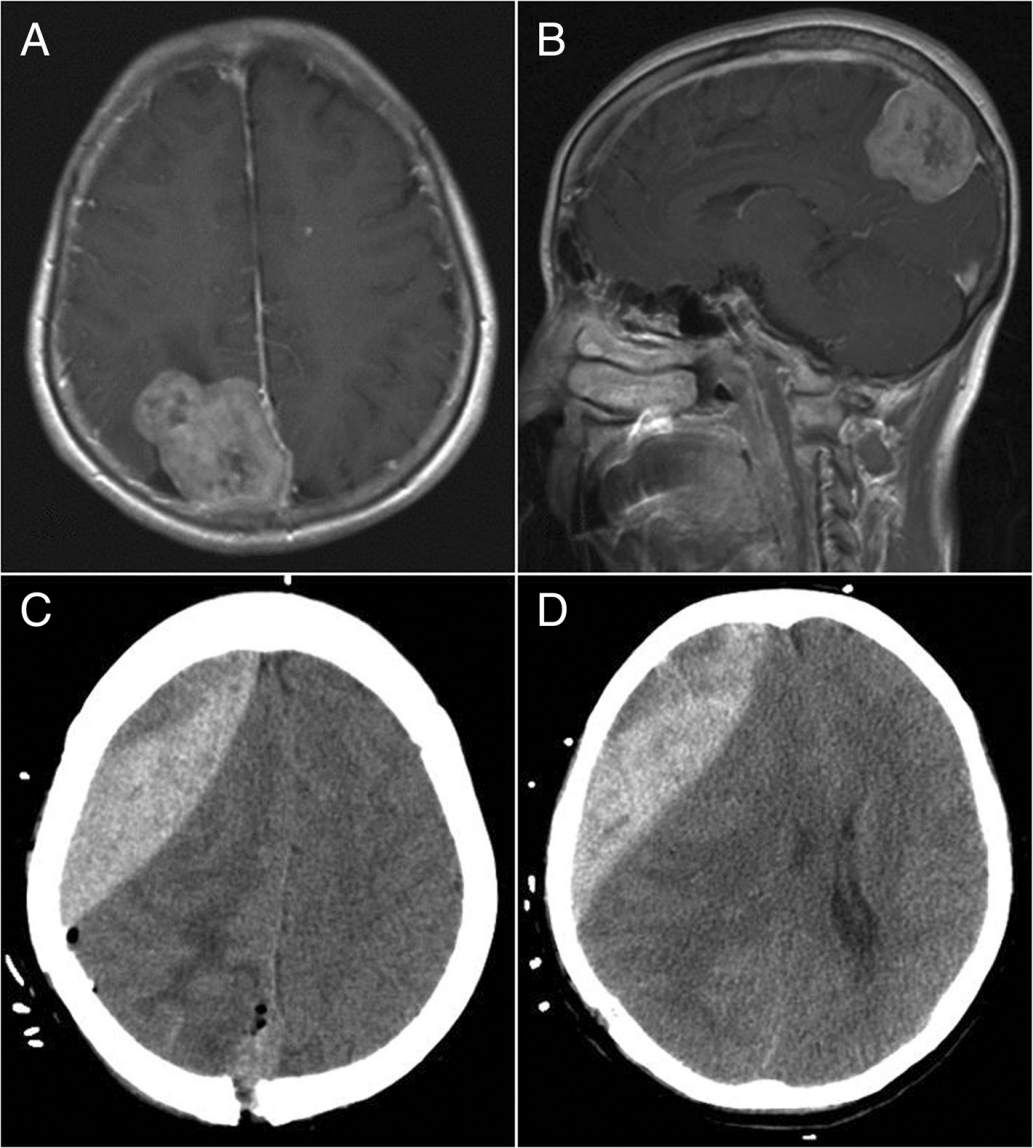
Ipsilateral remote hemorrhage in case 2. a, b Preoperative-enhanced MRI revealed a meningioma at the top right of the sagittal sinus. c, d CT showed an epidural hematoma in front of the surgical field after the tumor resection
